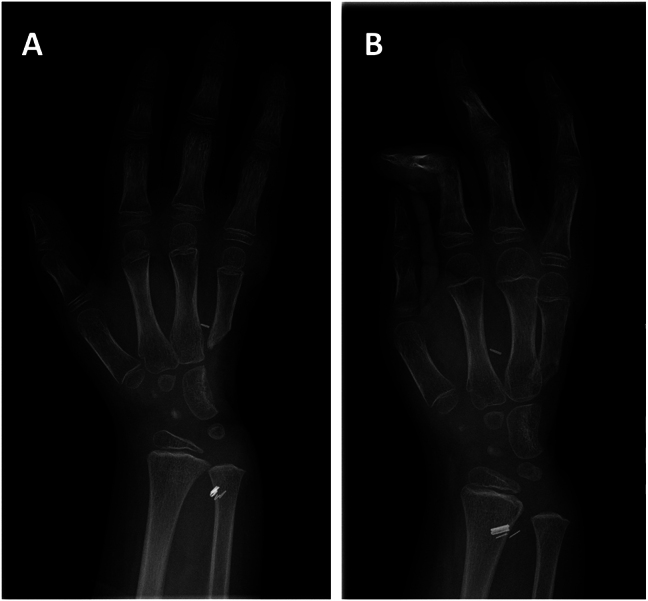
Postoperative radiography of the hand after tumor resection and primary closure; (A) anteroposterior view, (B) lateral view.
